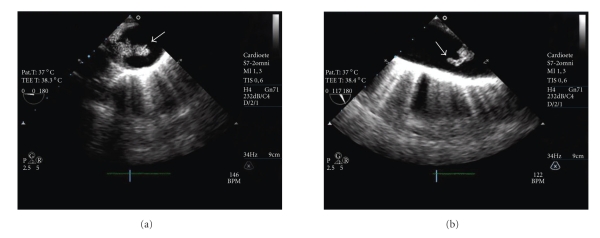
(a) Transesophageal echocardiogram (TEE) image of the proximal descending aorta (PDA) with sectioning plane at 0°. A large polypoid sessile vegetation (2.1 × 0.9 cm) attached to the intima can be seen at the beginning of descending thoracic aorta (*arrow*); (b) TEE image of the PDA with sectioning plane at 117°, showing the same vegetation (*arrow*).
